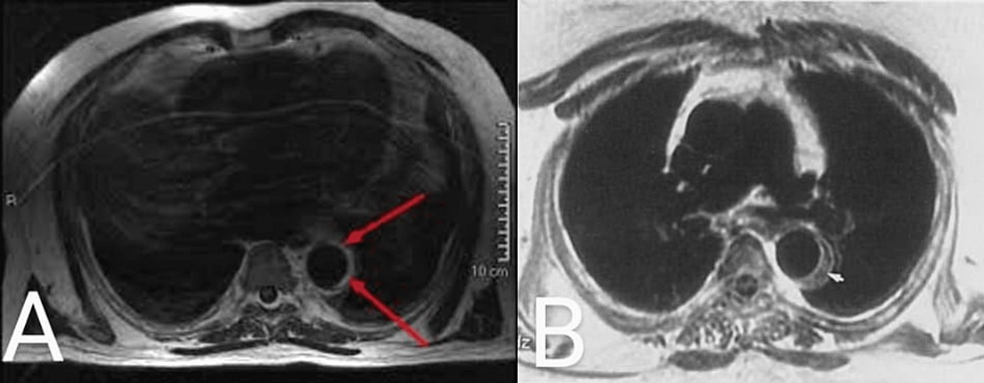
MRI showing increased signal intensity with diffusion restriction (A) T1-weighted image of cardiac MRI depicting blood flow in the descending aorta which is surrounded by the rim of bright material with increased echogenicity (red arrows), representing a subacute intramural hematoma. (B) T1-weighted cardiac MRI representing an intramural hematoma, surrounded with mixed hypo- and isodense rim (white cursor) suggesting a chronic duration.
